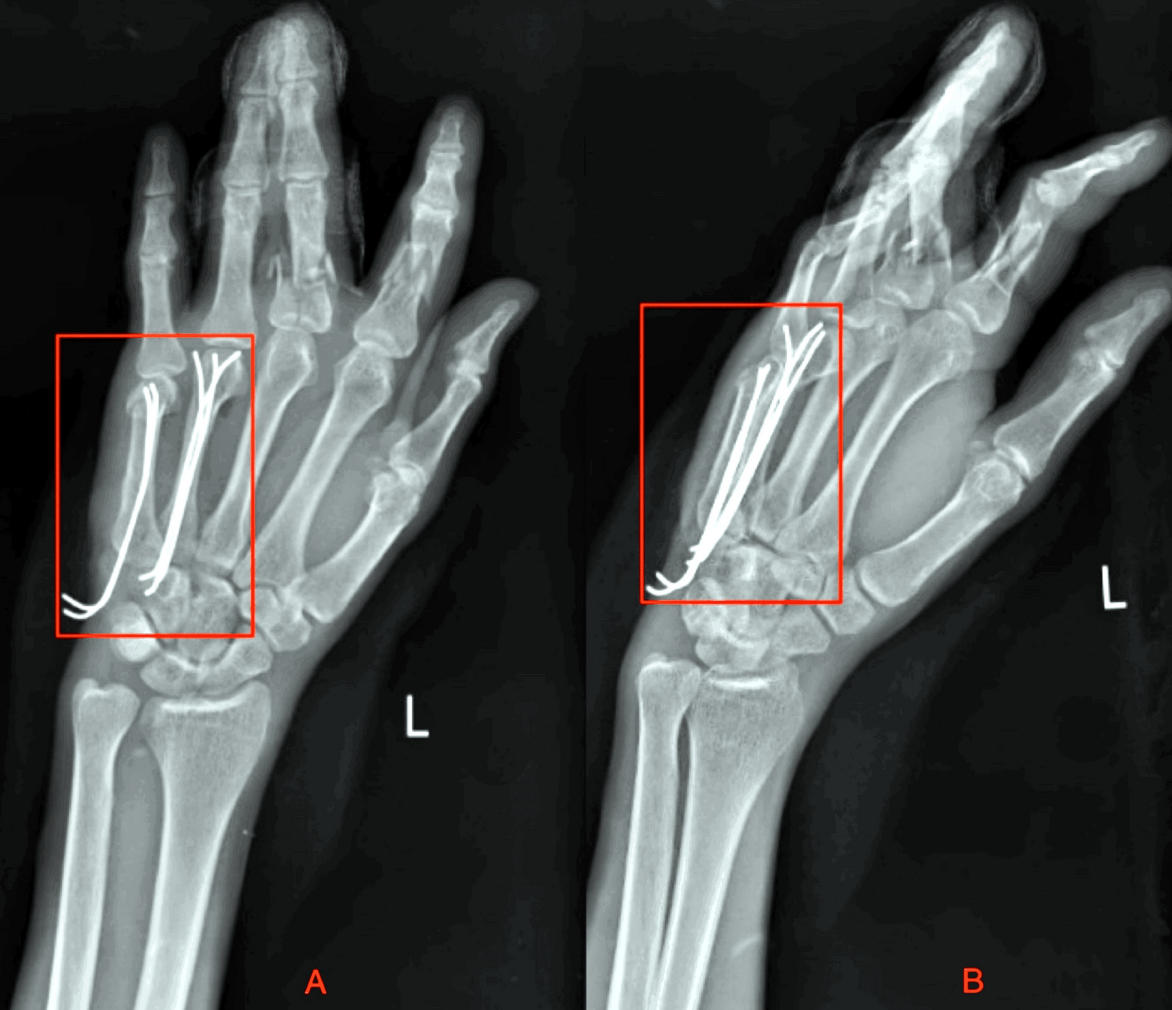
Post-operative X-ray of the left hand A) Antero-posterior view; B) Lateral view
